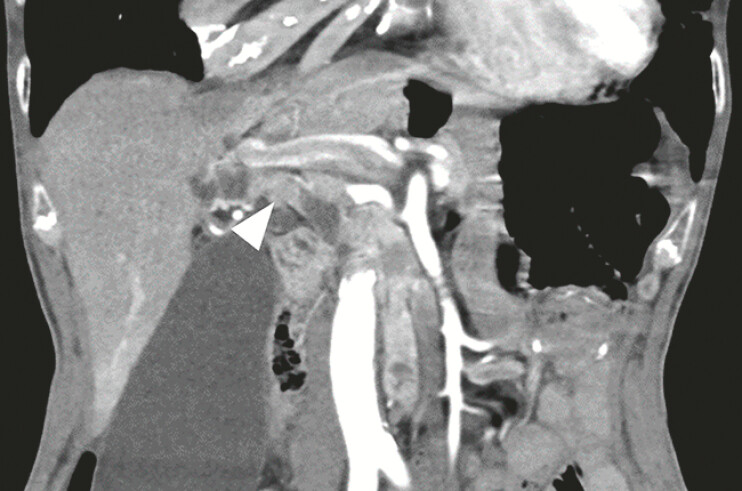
Hypervascular tumor in the common bile duct is revealed on the computed tomographic imaging.
